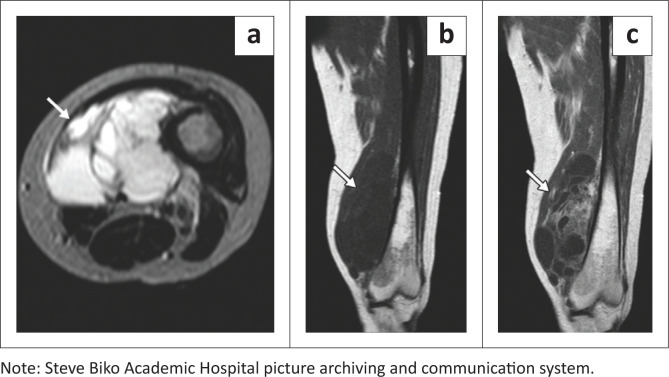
Axial T2-weighted images (a) of the left thigh confirm the intramuscular myxomas, which are T2 hyperintense with internal septations. Coronal T1 pre- and post-gadolinium images (b, c) demonstrate hypointense myxomas with heterogenous peripheral and patchy internal enhancement. The lesions are within the vastus medialis muscle.
